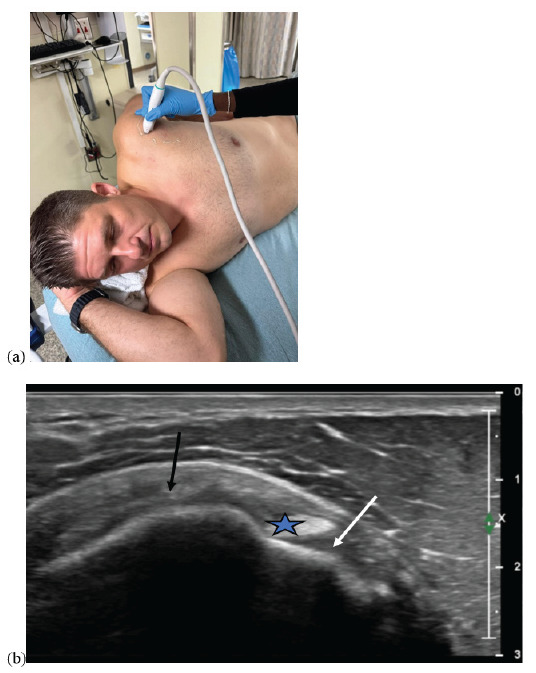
(a) Positioning of the ultrasound probe to obtain the transverse axis of the supraspinatus tendon using the supra-short protocol; and (b) normal transverse view of the supraspinatus tendon (black arrow). On this view, the biceps tendon (star) and subscapularis tendon (white arrow) are also visible (although not specifically assessed in the supra-short protocol).
